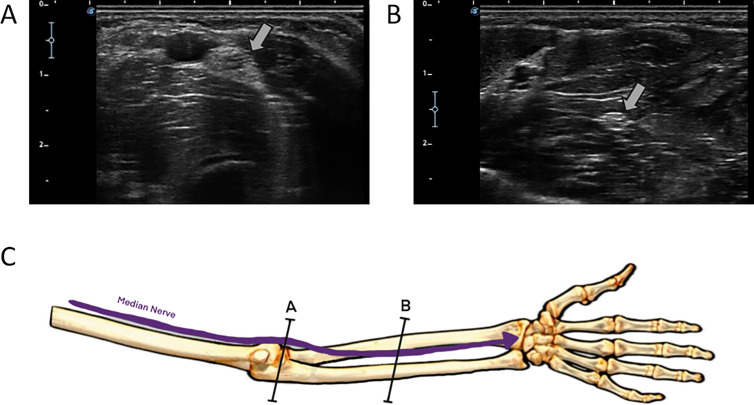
Ultrasound images of the MN at the proximal third (A) and middle third (B) of the forearm. The obtained images show the measurement sites used for morphometric analysis.
